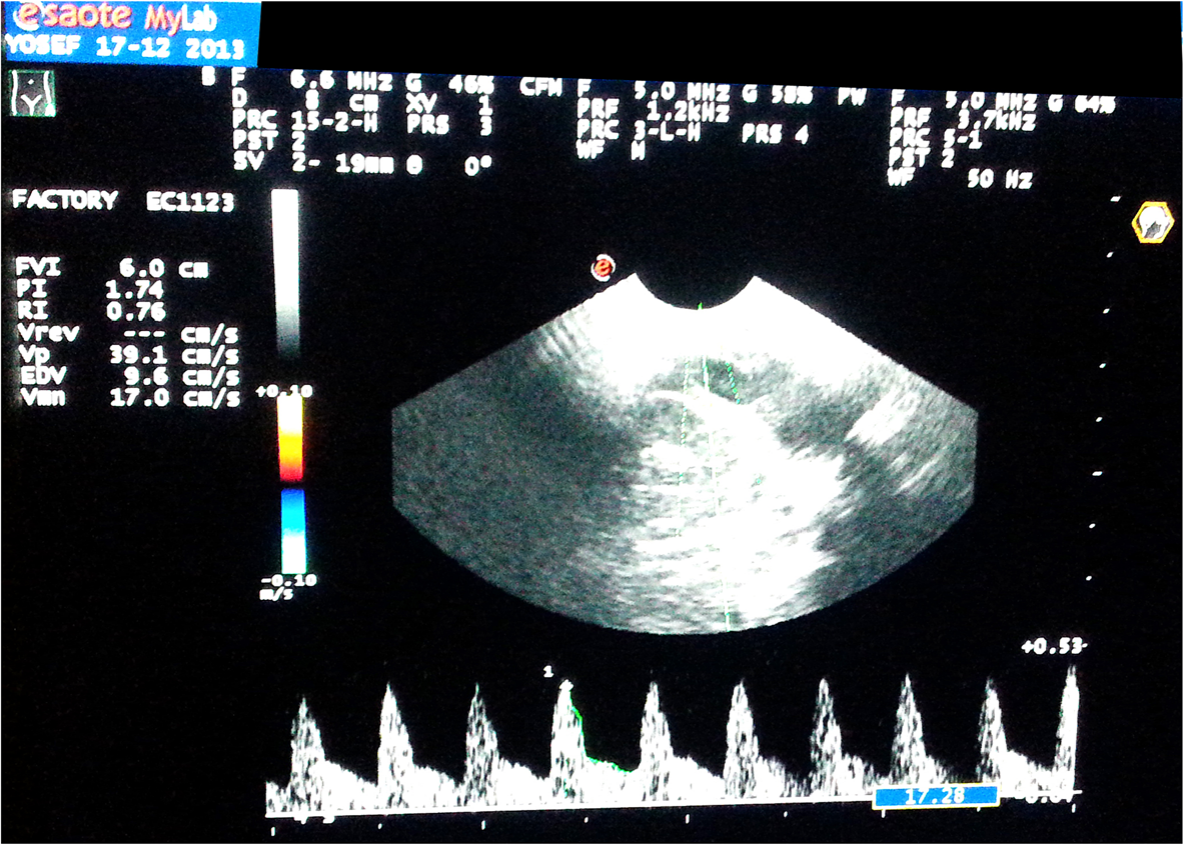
Colored Doppler Ultrasonography before KMC while the neonate in supine position.
